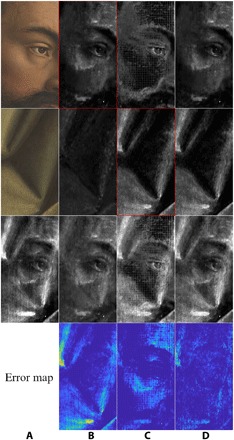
Results of the proposed algorithm applied to a detail from the *Adam* panel. Column (A) input data; (B to D) results from first (B), second (C), and combined (D) approach—the latter takes the best of both first and second approaches. Top row: Interior (*Adam*) side RGB input (before conservation) and various reconstructed x-ray images. Second row: Exterior (drapery) side RGB input (image mirrored for easier comparison with x-ray images) and the reconstructed x-ray images. Third row: Original mixed x-ray input image (left) and mixtures of the reconstructed x-ray images in rows 1 and 2. Bottom row: Visualization of the error map for each approach.
